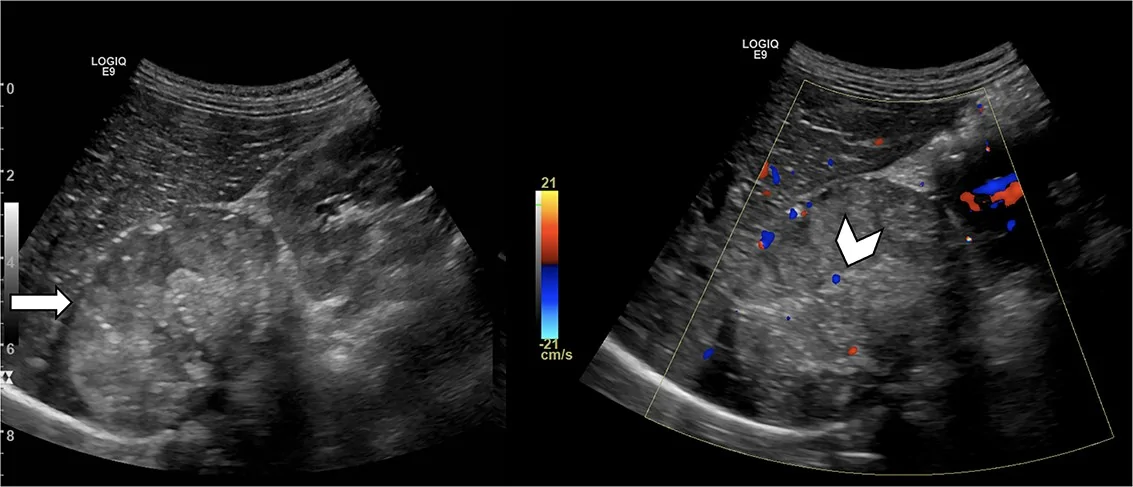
Sagittal ultrasound image demonstrates a 5 cm heterogenous right adrenal mass (arrow) with mild vascularity (arrowhead).
